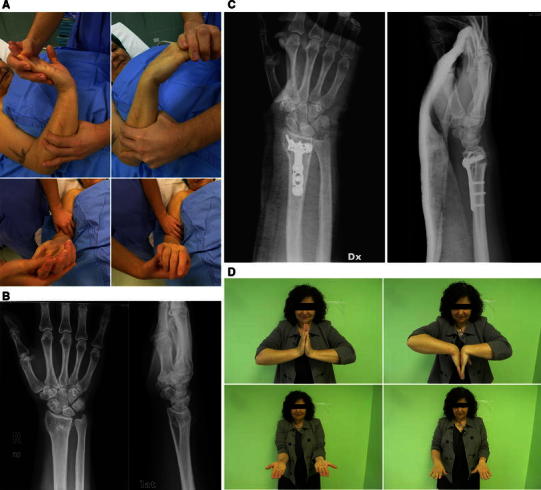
Case 2. a Range of motion evaluation before surgery with significant reduction of flexion. b Preoperative X-rays showing the dorsal inclination of articular surface of the distal radius. c Postoperative X-rays showing the correction of the dorsal deformity achieved. d Good functional recovery at 2 months after surgery
